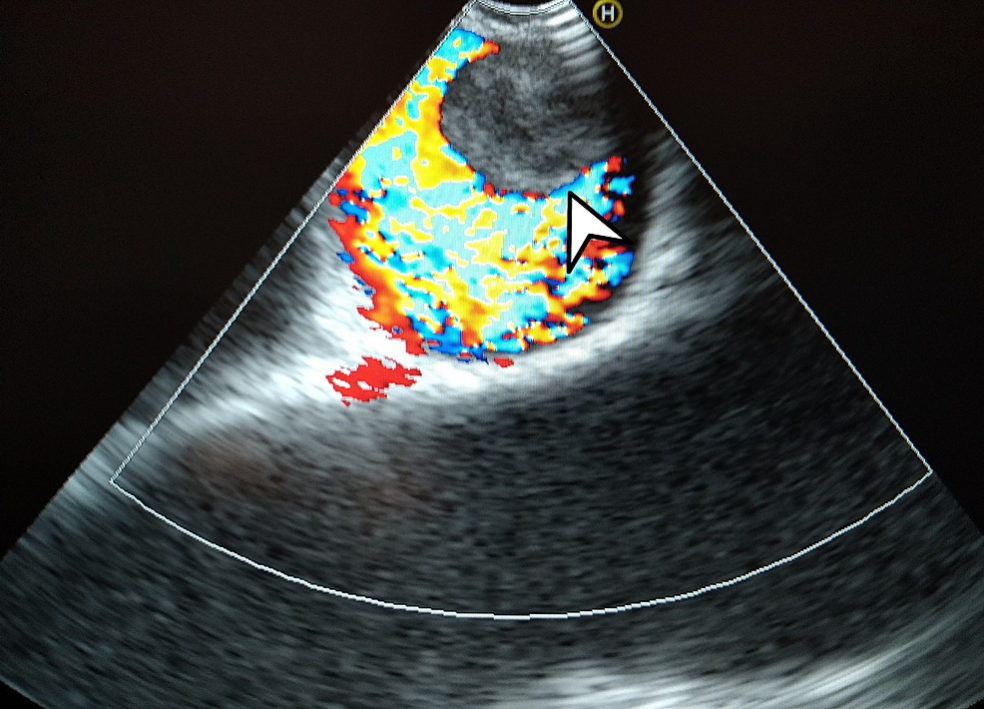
Color Doppler showing the blood flow around the intravascular tumor
